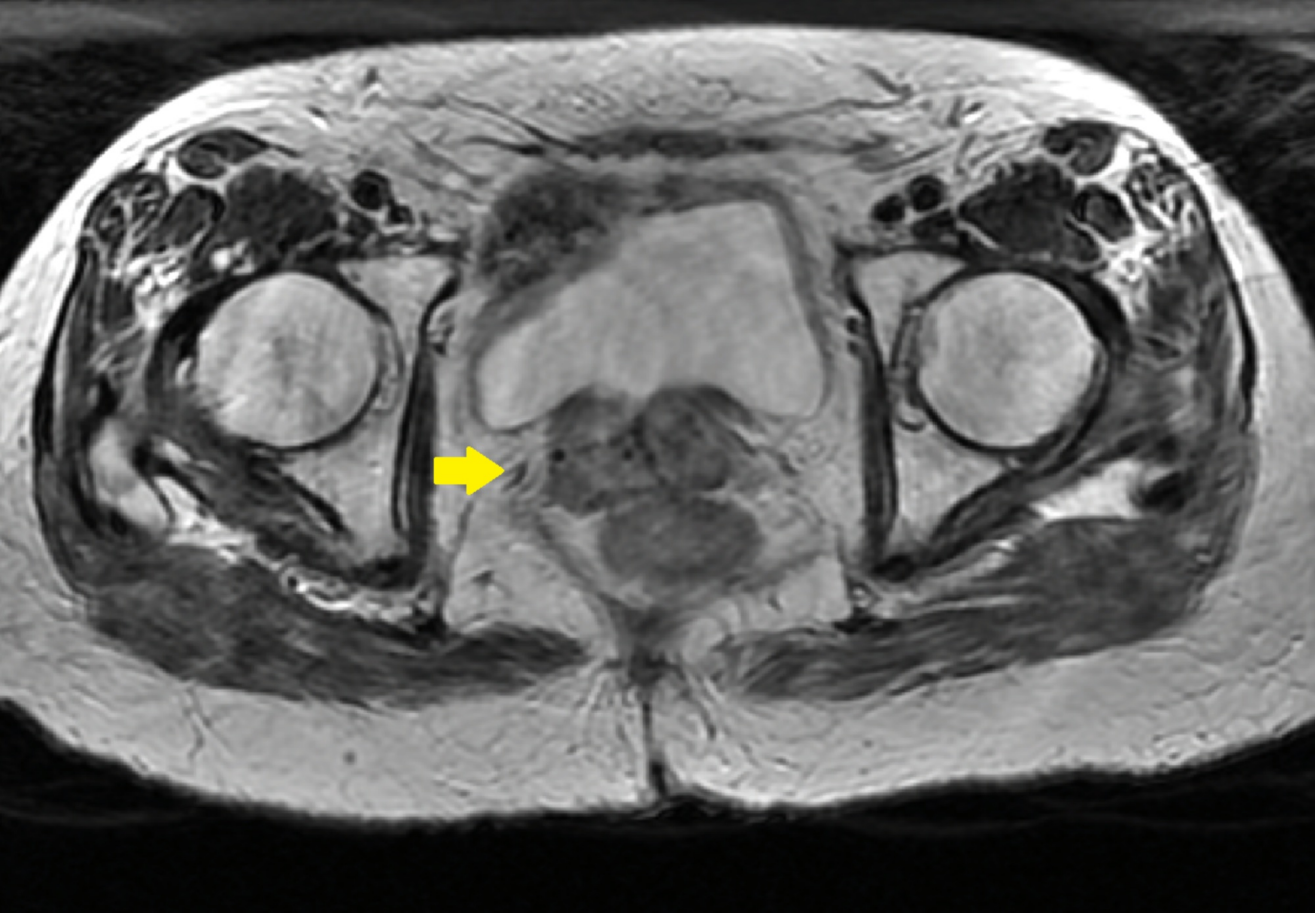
Pre-implant axial magnetic resonance image showing vaginal cuff recurrence
